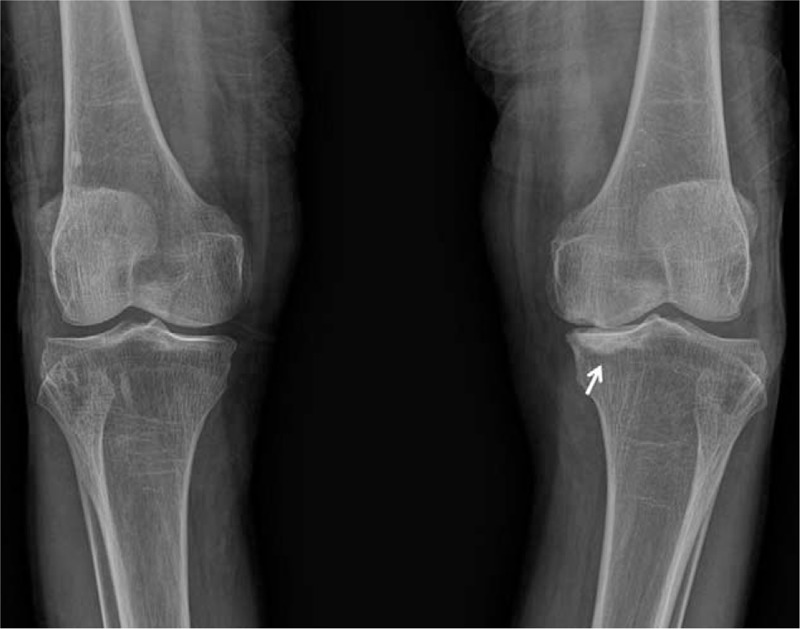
AP plain radiograph of the knee 2 months after intra-articular corticosteroid injection showed a new radiodense lesion (arrow) relevant to the ON of the medial tibial plateau.
